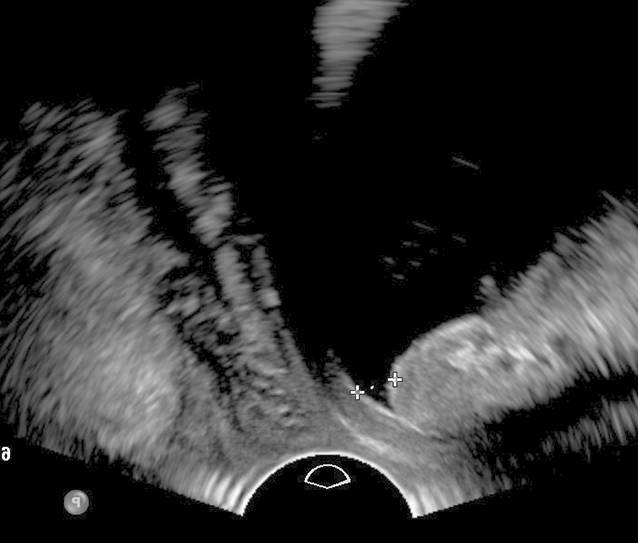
Vor einer geplanten chirurgischen Deszensusoperation sollte mithilfe des Ultraschalls eine Pathologie des Uterus und der Adnexen ausgeschlossen werden. Dies ist insbesondere bei einer geplanten uteruserhaltenden Operation von Bedeutung. Bei Vorliegen eines Descensus uteri kann eine präoperativ durchgeführte Sonographie eine Elongatio colli detektieren, welche bei einem organerhaltenden Vorgehen postoperativ zu einer Persistenz der Symptome führen kann [2]. Ebenfalls sollte präoperativ sonographisch das Risiko für das Bestehen einer lavierenden Inkontinenz evaluiert werden. Unter einer lavierenden Inkontinenz versteht man eine Inkontinenz, die durch einen Prolaps maskiert wird. Bei Belastung kommt es durch einen rotatorischen Descensus urethrae zu einem Abknicken der Urethra, sodass eine bereits vorhandene Inkontinenz kaschiert wird. Nach einer operativen Korrektur des Deszensus kann folglich die bereits präoperativ bestehende Inkontinenz symptomatisch werden [12].
